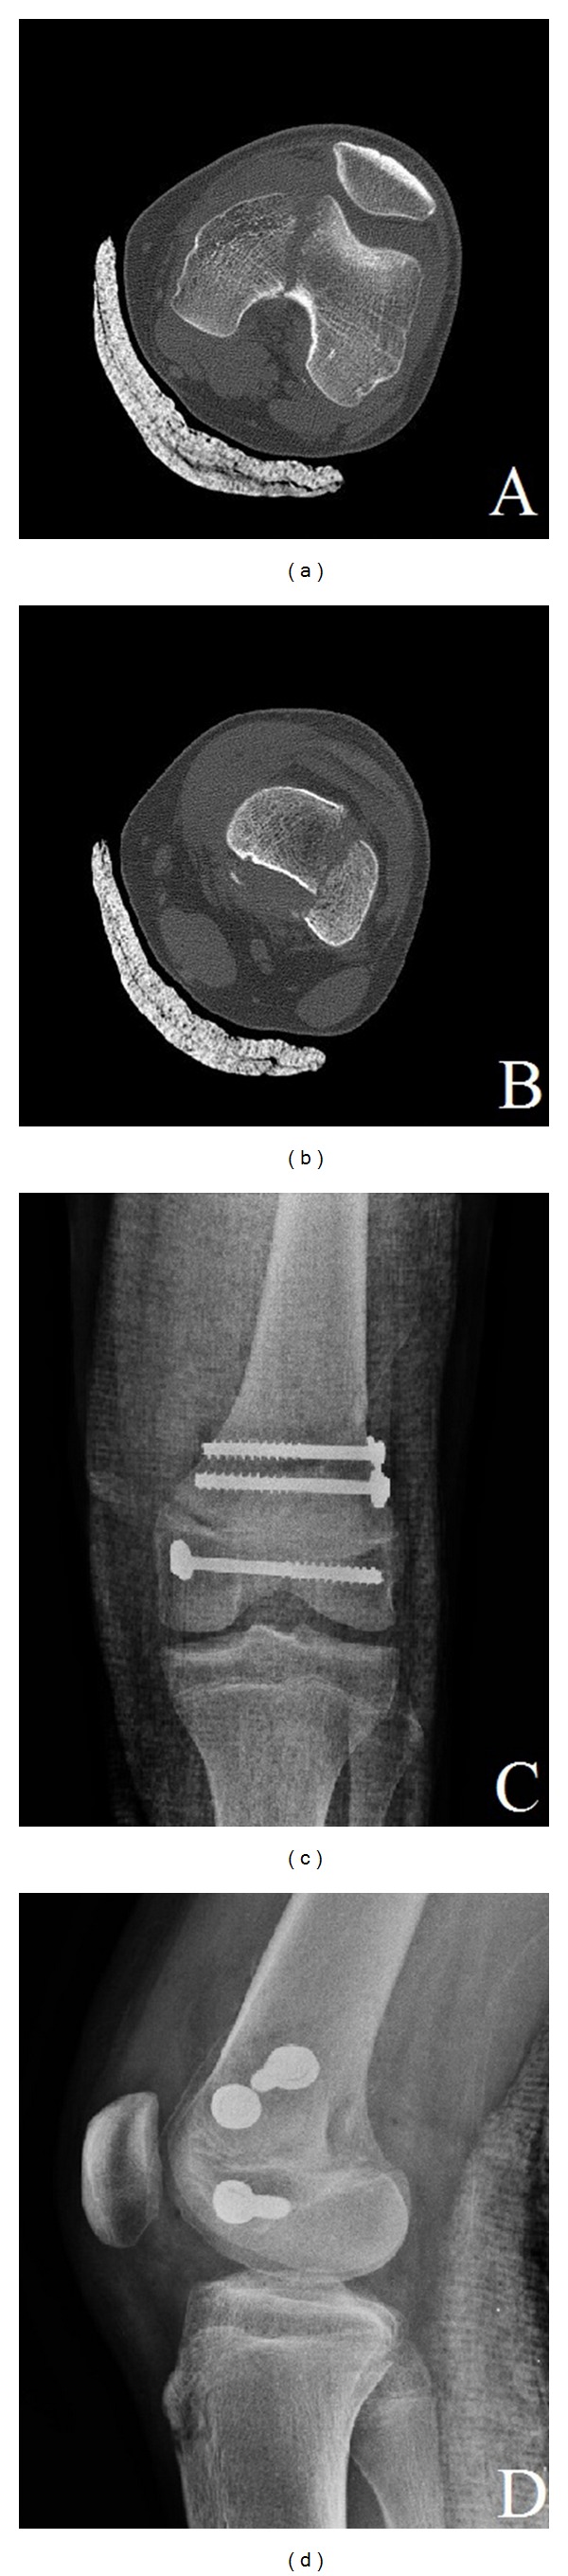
Computed tomography of physeal (a) and metaphyseal (b) fracture. Postoperative anteroposterior and lateral radiograph of the left knee (c-d).
